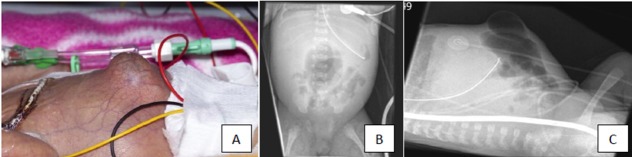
Fig. 1(A): Photograph of case 1 on day 23 of life, showing right peri-umbilical swelling;(B): AXR of Case 1 on day 23 of life showing localized gas shadow at the site of peri-umbilical swelling; (C): Lateral x-ray of the abdomen of case 1 on day 23 of life showing dilated bowel and air filled cyst at the site of peri-umbilical swelling.
